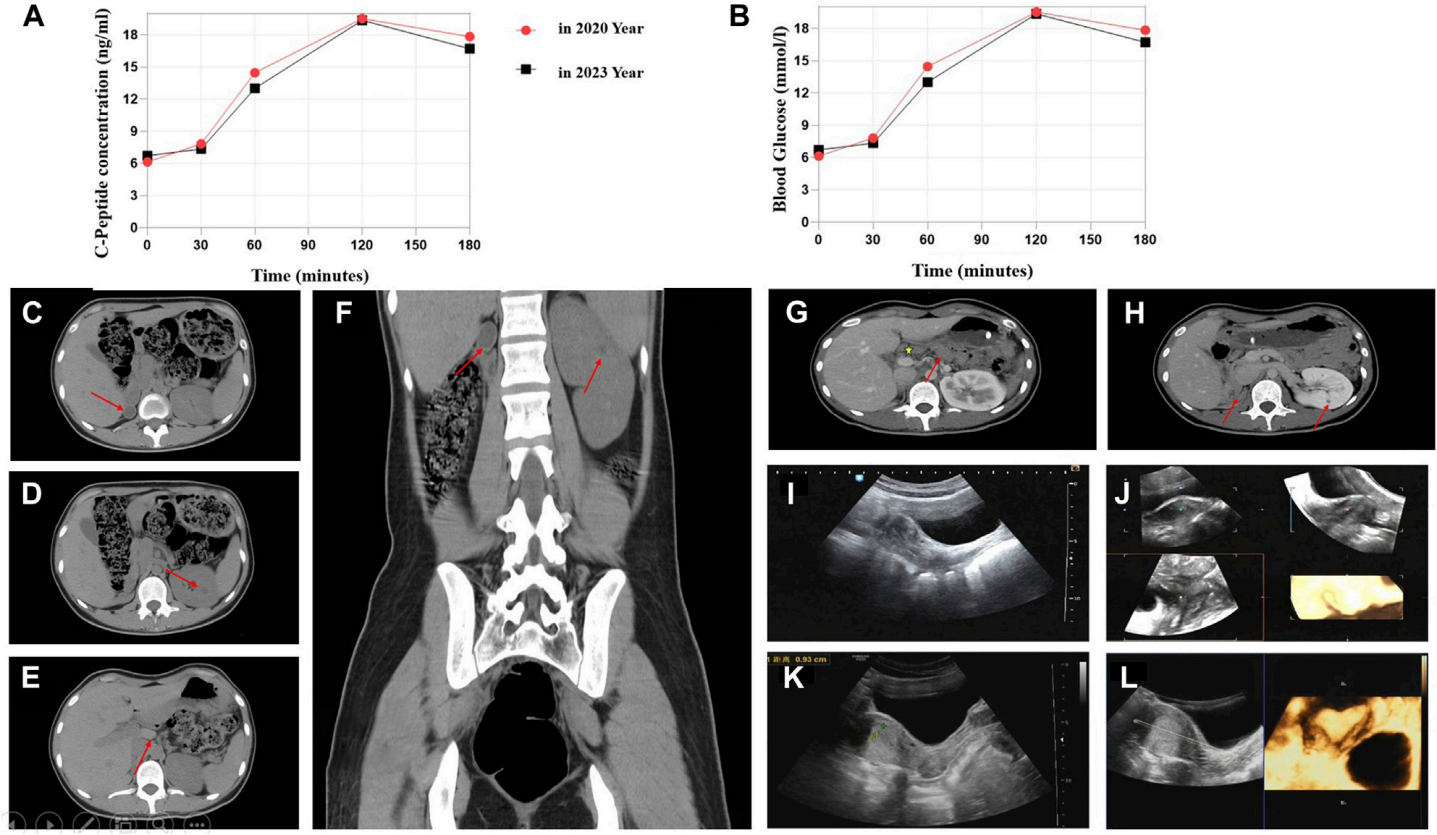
C-Peptide test, OGTT test, and imaging findings. (A) C-peptide concentration after glucose overload in 2020 and 2023 year. (B) Oral glucose tolerance test (OGTT) in 2020 and 2023. Abdominal CT image: (C) Right atrophied kidney (arrow). (D) Multiple small cysts in the left kidney (arrow). (E) Abnormal morphology of the pancreas with the atrophied tail part (arrow). (F) Enlargement of the left kidney. Contrast-enhanced CT scan: (G) Abnormal pancreas (arrow represents the pancreas body and atrophied tail). (H) Small cysts in the left kidney. Ultrasonographic view of the uterus. (I,J) Depression at the base of the uterine cavity with 0.6-cm uterine myometrial fundus projecting into the uterine cavity (2020 year). (K,L) Bowed uterus imaging result (2023 year).
